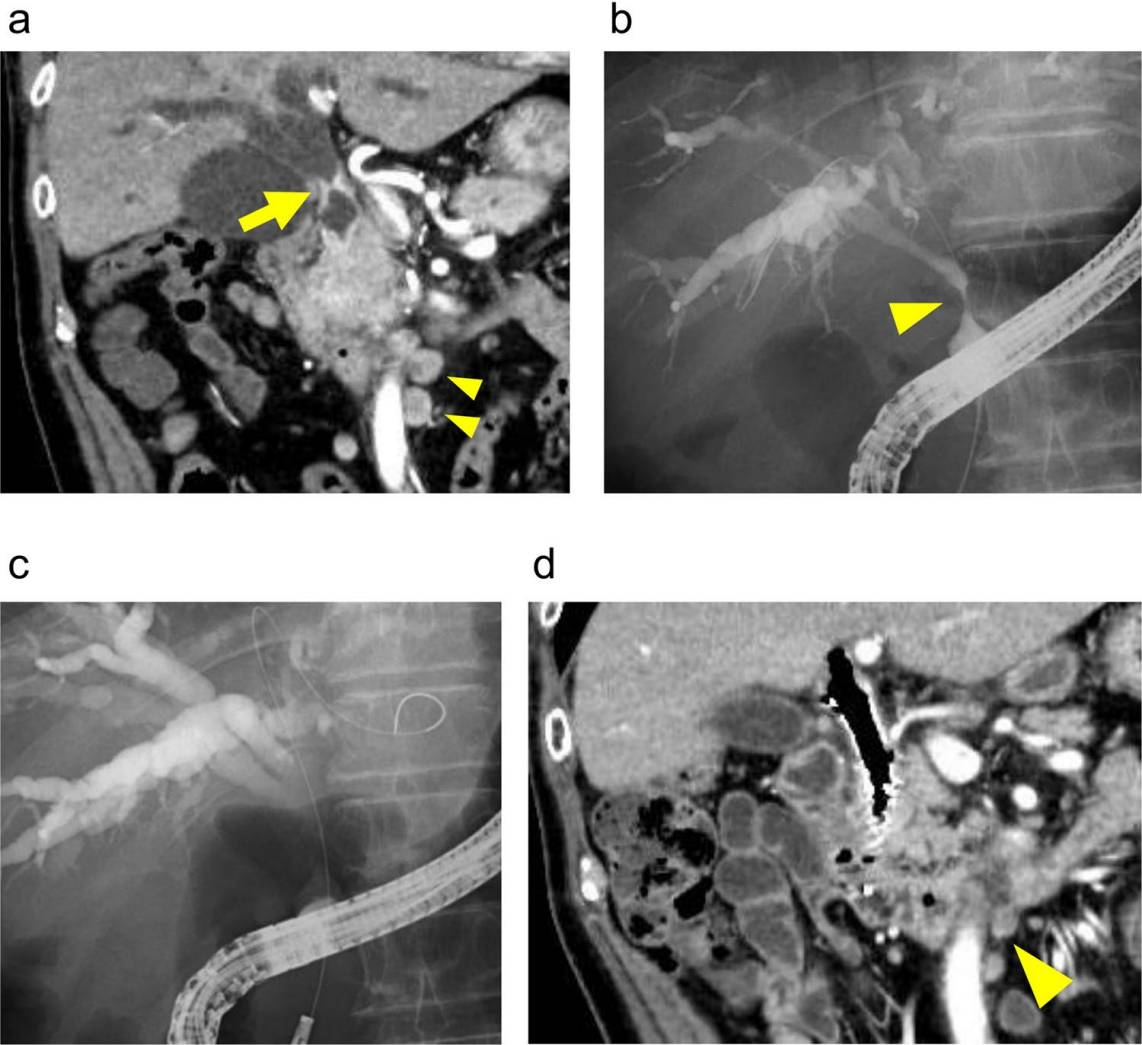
Preoperative abdominal MDCT and images of ERCP. a An abdominal MDCT showed a thickening common bile duct (arrow) and swollen para-aortic lymph nodes (arrowheads). b Narrowing of distal bile duct was seen in the first ERCP (arrowhead). c A guidewire was inserted in the left hepatic duct and the common bile duct during the first ERCP. d An abdominal MDCT after six courses of chemotherapy showed shrinkage of para-aortic lymph nodes (arrowhead). *MDCT* multidetector computed tomography, *ERCP* endoscopic retrograde cholangiopancreatography
